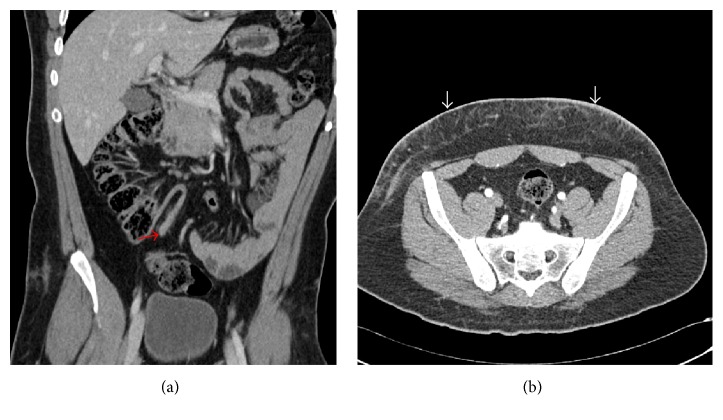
(a) CT abdomen, coronal view, showing inflamed appendix (red arrow). (b) CT abdomen, transverse view, showing subcutaneous fat stranding of lower anterior abdominal wall (white arrows).
